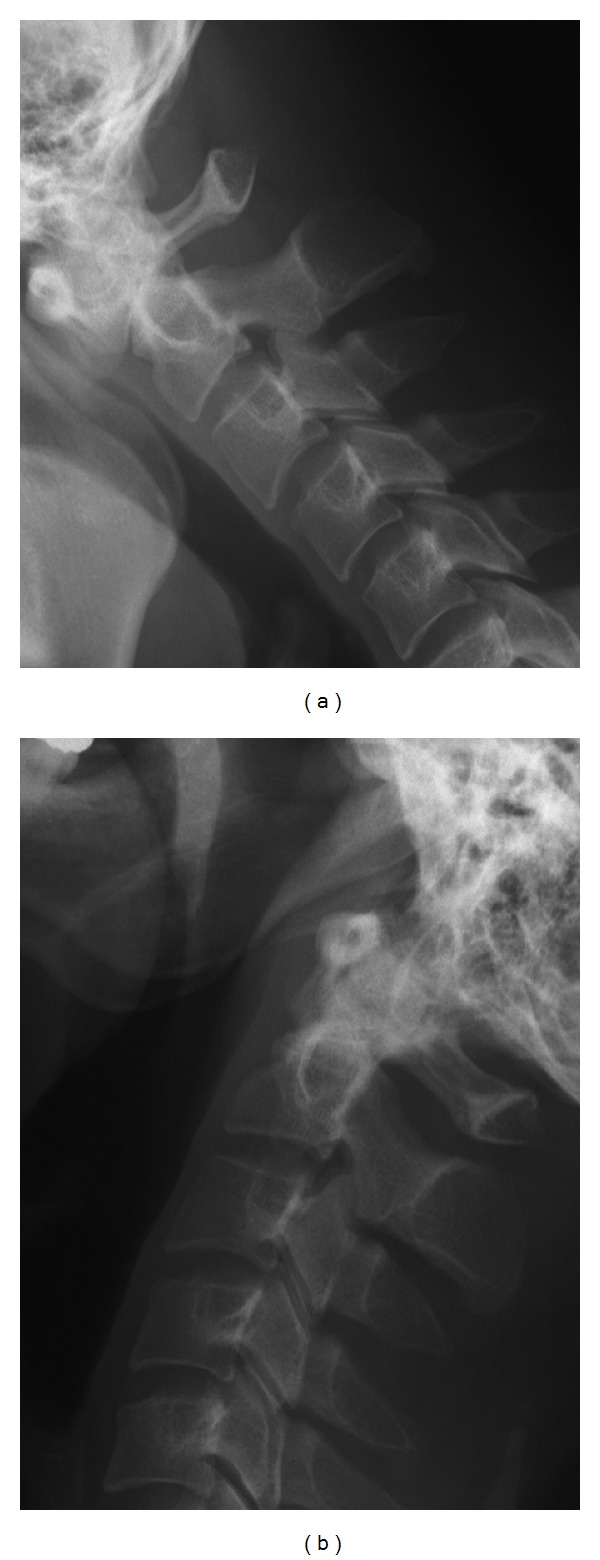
Plain X-ray images at the first visit under (a) flexion and (b) extension. Instability of the atlantoaxial joint was observed, with ADI increasing to 8 mm under flexion and reducing to ≤3 mm in extension.
